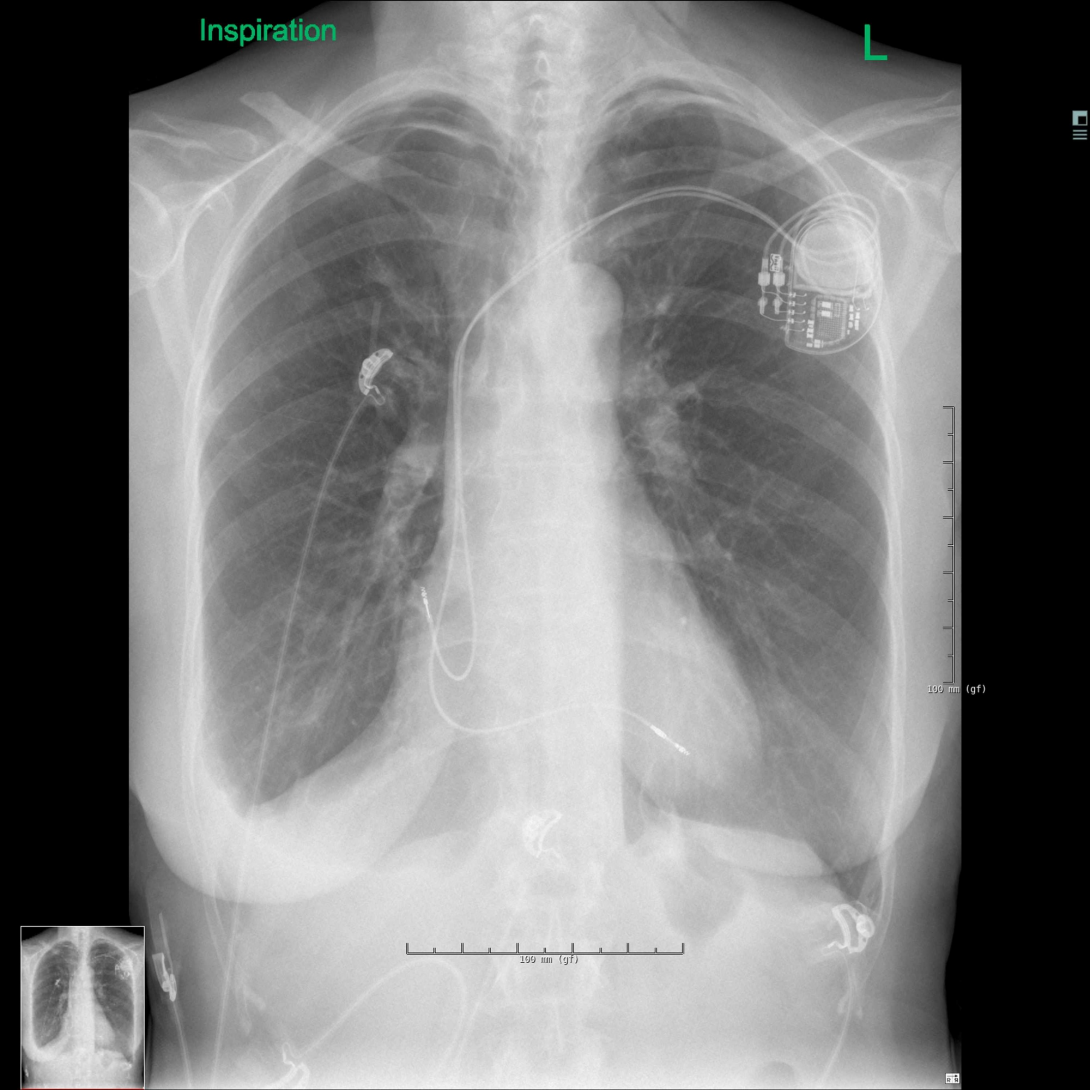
Chest X-ray during inspiration on day 3.
